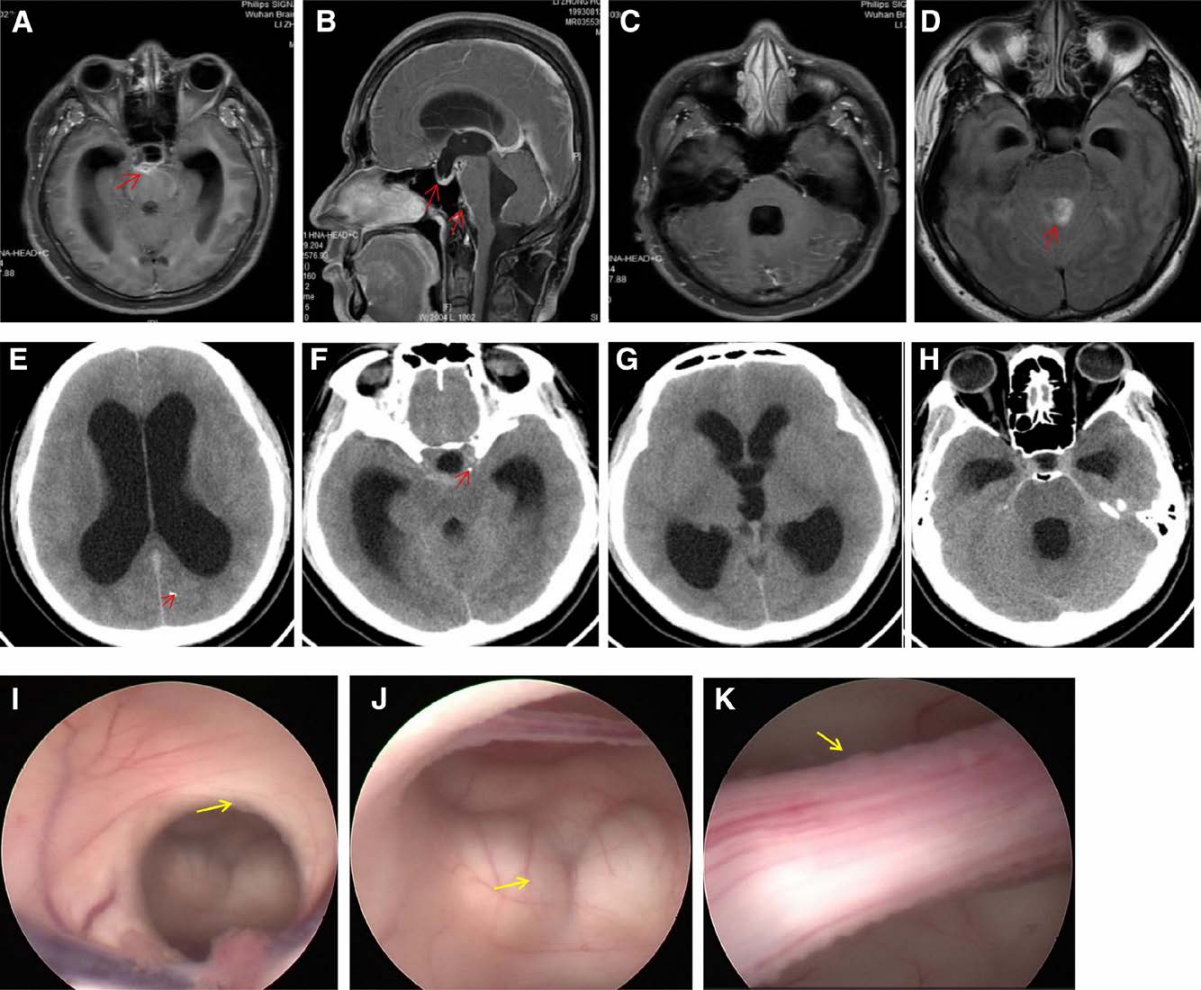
Preoperative neuroimaging and intraoperative ventriculoscope exploration. On admission, MRI showed: (A) inflammation and adhesion of the basal cisterns with enlarged lateral ventricles. (B) Enlarged third ventricle with downward herniation and basal cistern inflammation. (C) Enlarged fourth ventricle. (D) Heterogeneous signals within ventricles (FLAIR imaging). Three weeks later, head CT revealed (E) calcified lesions in the left occipital lobe, enlarged lateral ventricles, and shallow sulci. (F) Calcified lesions in the parasellar region. (G) Enlarged lateral and third ventricles. (H) Enlarged temporal horns and the fourth ventricle. Intraoperative ventriculoscopy showed (I) white, fine granular, and membranous proliferations covering the ventricular edges. (J) The mammillary bodies are covered by inflammatory proliferations obscuring the basilar artery. (K) Enlarged interpeduncular fossa with granular proliferation. CT = computed tomography, FLAIR = fluid attenuated inversion recovery, MRI = magnetic resonance imaging.
